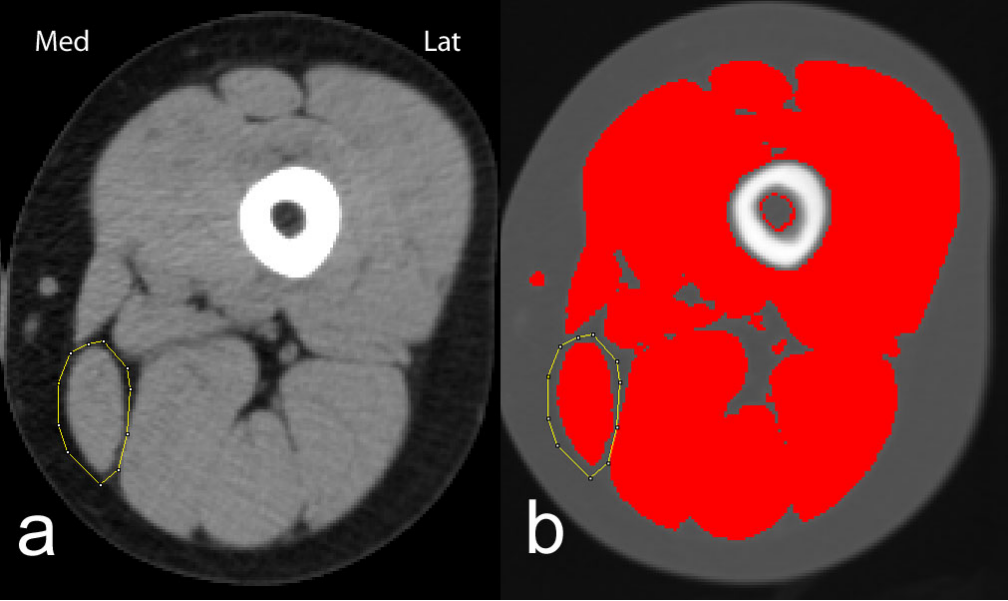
CT image of left thigh viewed with ImageJ. Musculus gracilis encircled. a) with level 50 HU and width 400 HU used when encircling the muscle and b) after highlighting areas with attenuation between 1 and 101 HU.
